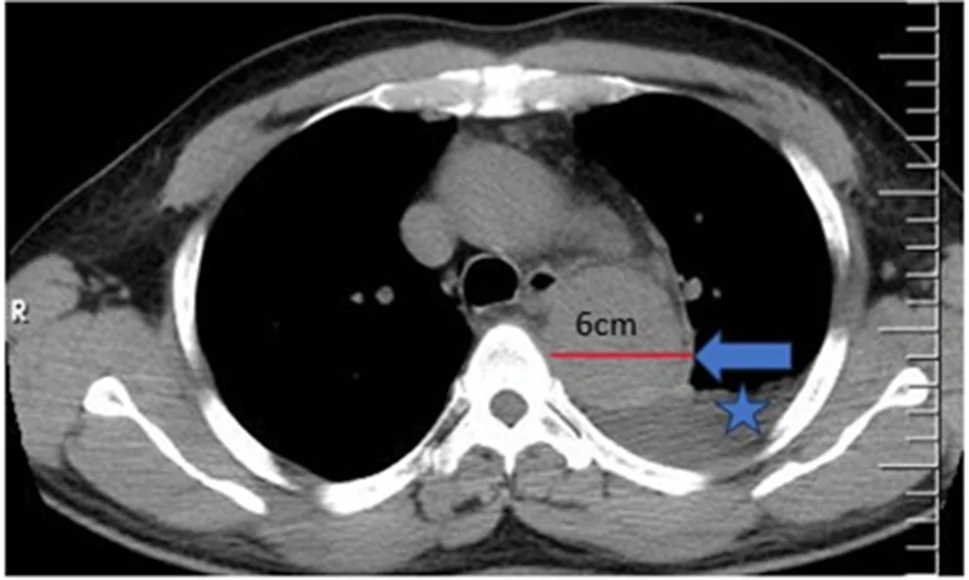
Pre-contrast chest CT showing the descending thoracic aorta at its widest point (arrow), measuring approximately 6 cm in diameter, and a left-sided pleural effusion (star)
